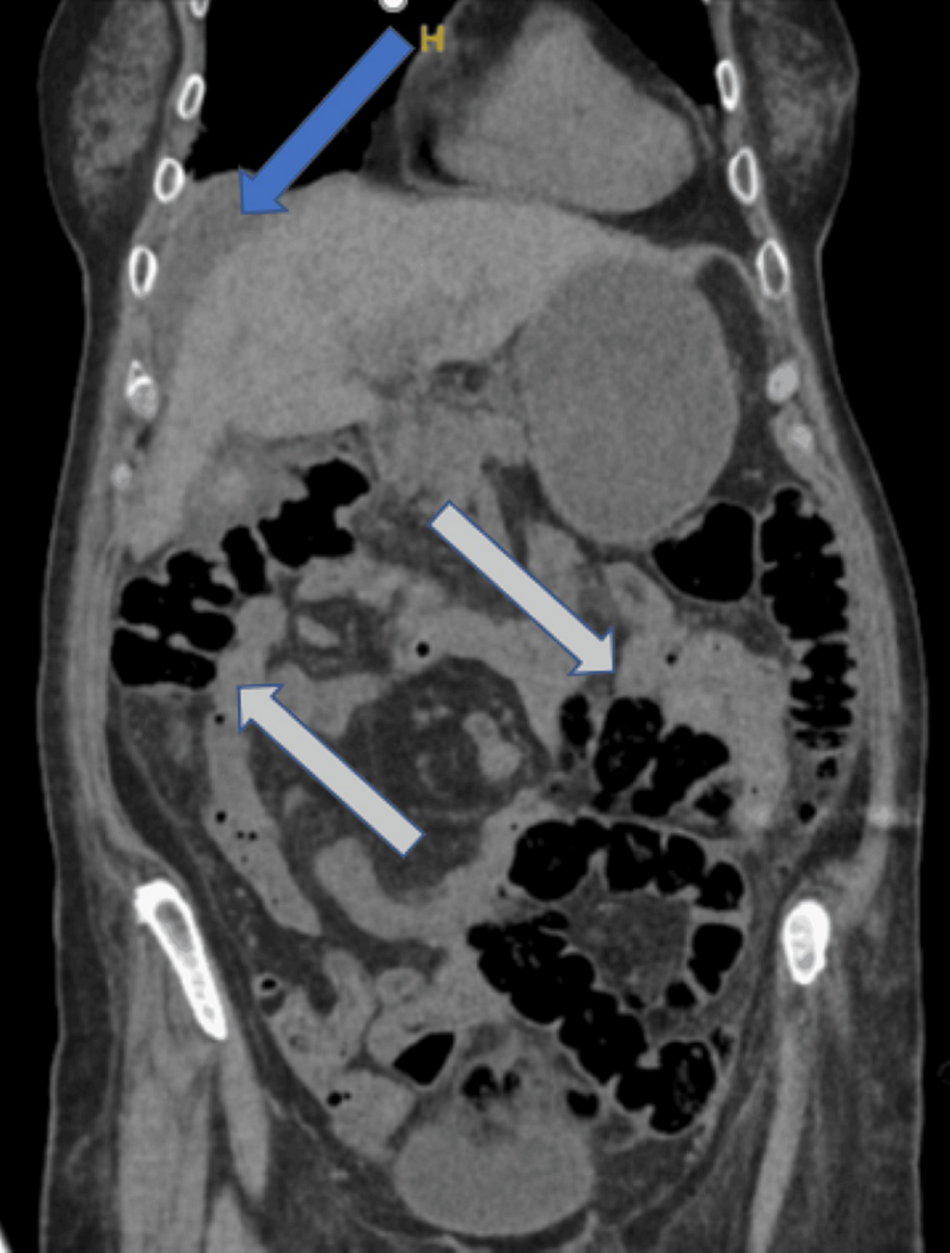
CT without contrast of the abdomen/pelvis (coronal view). Radiologic impression read as residual moderate intra-abdominal ascites.
